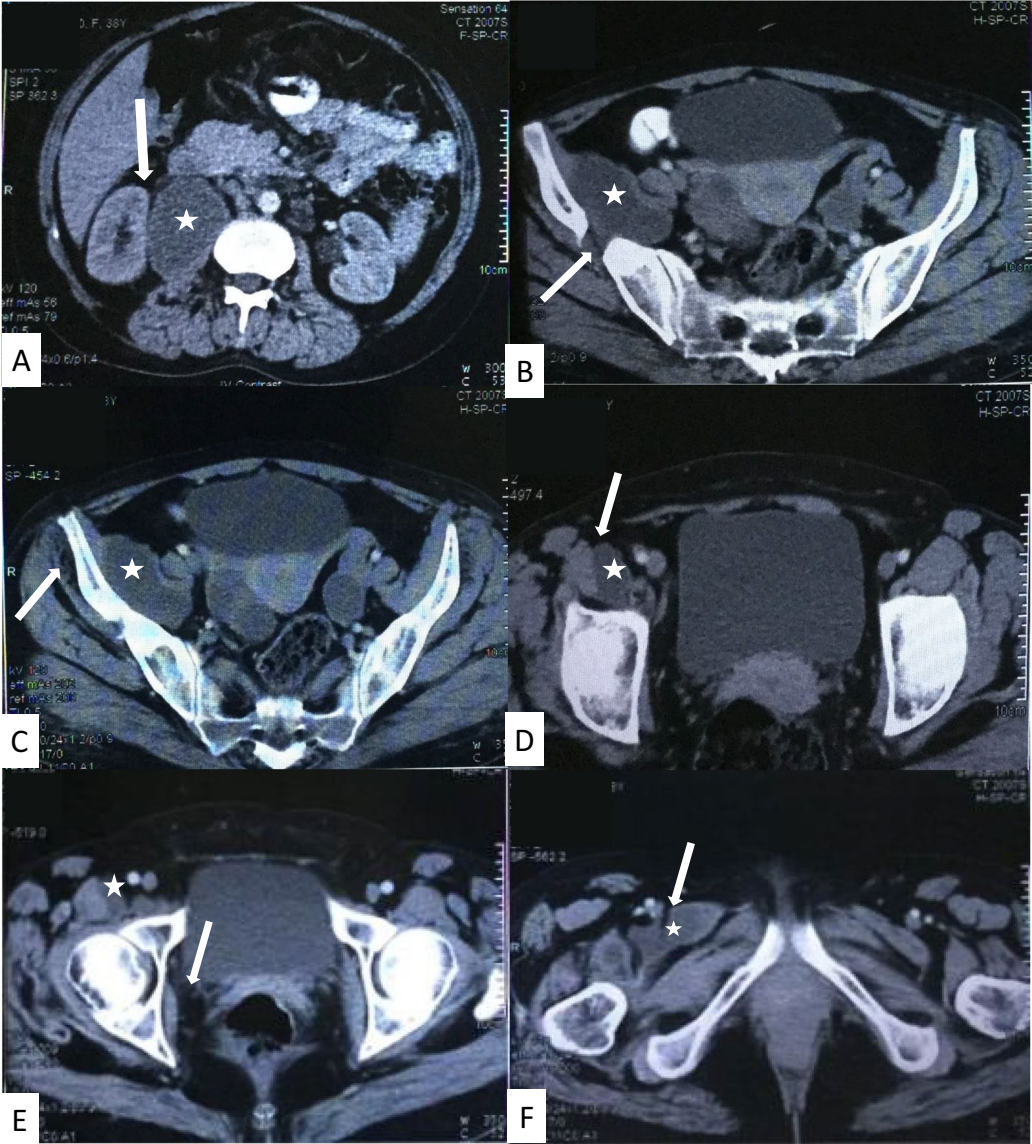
Abdominal and pelvic CT scan showing a transverse section in the Iliopsoas abscess (white star) with (A) the right kidney slightly pushed anteriorly (white arrow), (B) necrosis of the right ileum wing (white arrow), and (C) abscess in the right gluteal muscles (white arrow). The abscess extended to the right piriformis muscle (white arrow) (D) down to the level of the right obturator muscle (white arrow) (E), and the right femur adductor muscle (white arrow)(F)
